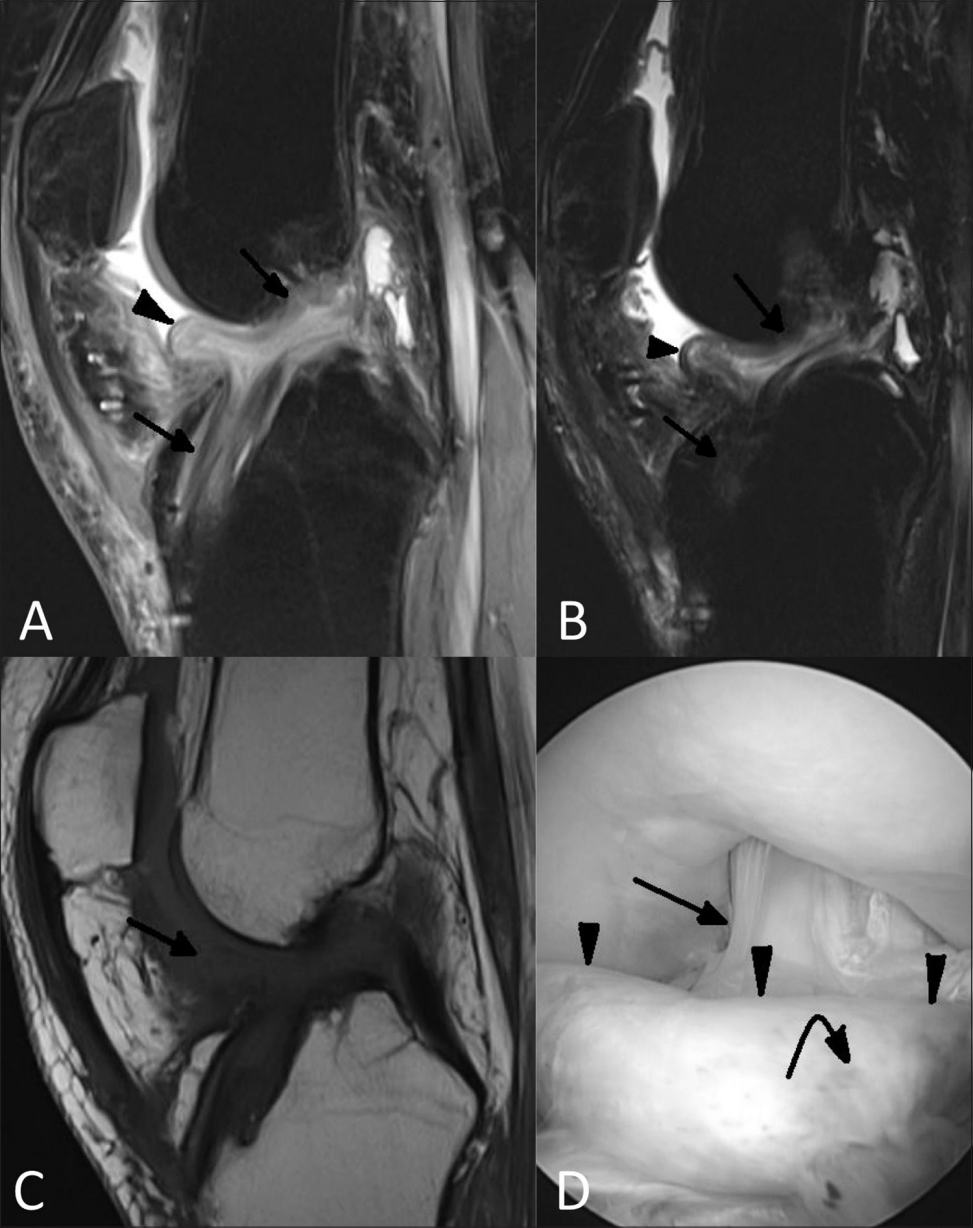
(A-D) Case 1: Fat-suppressed sagittal proton-density weighted (A) and T2W (B) MRI images show an anterior cruciate ligament (ACL) graft (arrow) with a hypointense nodule (arrowhead) attached to its anterior surface in the intercondylar notch. Sagittal T1W MRI image (C) shows the hypointense nodule (arrow) to be indistinguishable from the synovial fluid. Arthroscopic image (D) shows the cyclops lesion (arrowheads) attached to the ACL (arrow) with a head-like appearance, showing a focal area of discoloration resembling an eye (curved arrow)
